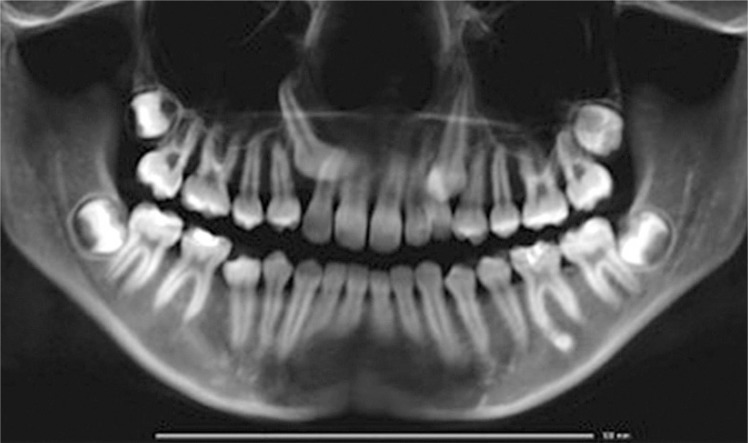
Panoramic reconstruction from CBCT.
